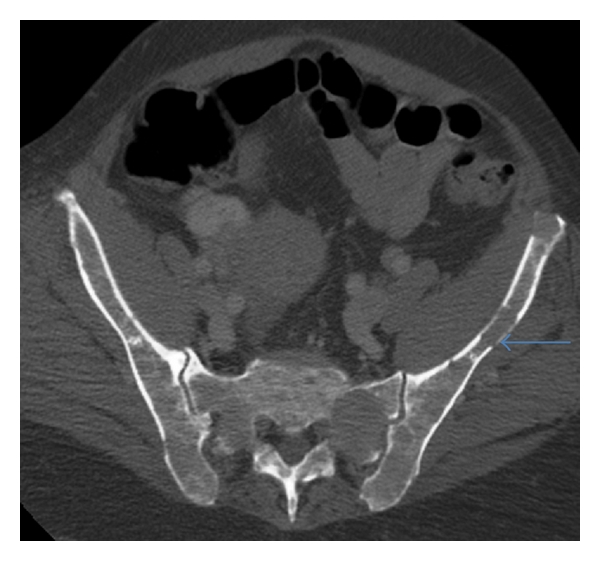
Axial CT pelvis: diffuse myeloma involving the sacrum and iliac bones bilaterally, with cortical destruction of the left iliac bone (arrow).
